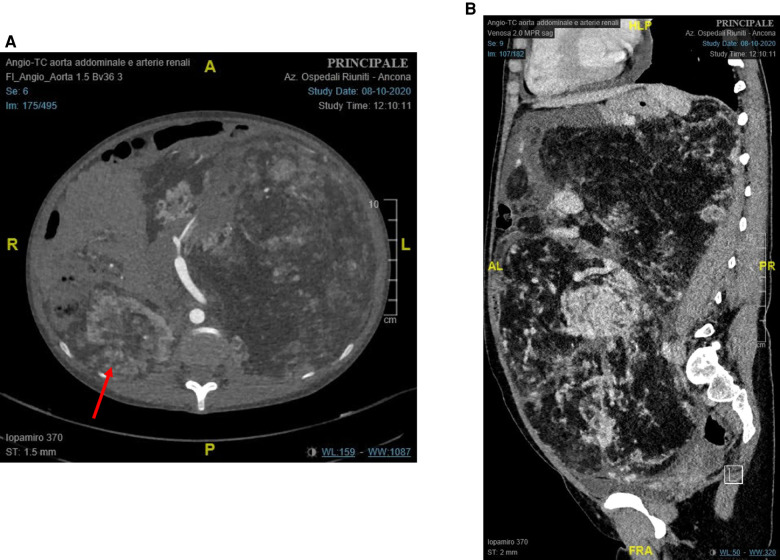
Preoperative contrast-enhanced computed tomography of the abdomen: (A) axial plane (red arrow: right renal angiomyolipoma); and (B) sagittal plane.
